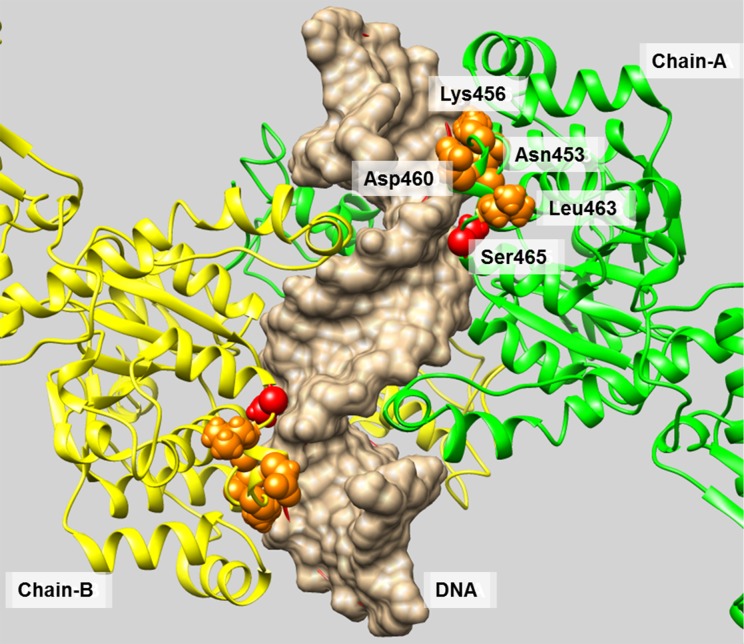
Structural model of FTL_1547 in its dimeric conformation (chains A and B) complexed with DNA. A mutation position Ser465 is colored in red. Ser465 is located next to positions 463, 460, 453 and 456 (colored in orange) that correspond to 471, 468, 464 and 461 from the X-ray structure (PDB chain 4i3h_A) described in [32] as critical functional positions located in the helical region of the C-gate facilitating DNA (T-segment) release.
